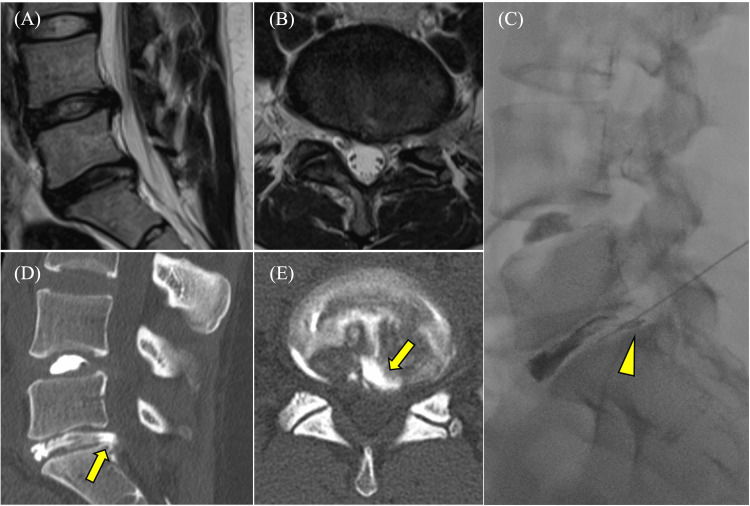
Magnetic resonance imaging, discography, and computed tomography myelography before the second surgery. Sagittal (A) and axial (B) T2-weighted magnetic resonance imaging taken before the second surgery showing near disappearance of the lumbar disc herniation but continued presence of a small high-intensity zone on the dorsal side at the L5/S1 disc. Discography (C) at L4/L5 showing normal findings and at L5/S1 showing a suspected annular tear (arrowhead); this was followed by disc block injection at the L5/S1 level. Sagittal (D) and axial (E) computed tomography myelography showing the same findings as on discography and pooling of the contrast medium inside the dorsal annulus (arrow).
